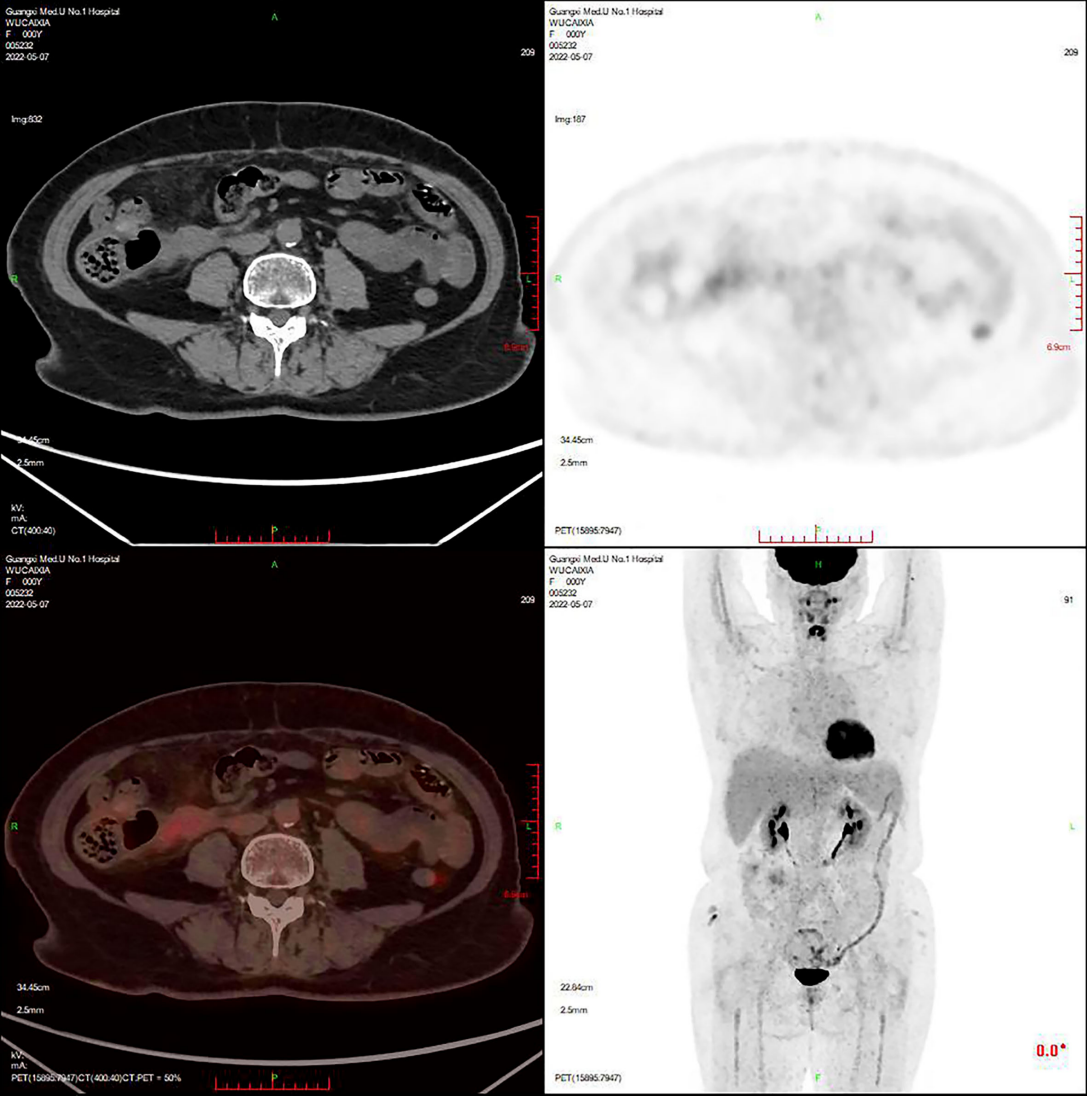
PET/CT. After chemotherapy and targeted treatment of lymphoma: (A) The right anterior lymph node lesions of the protodiaphragm have been absorbed and inactivated. (B) The primary ileocecal, colon, and mesenteric lesions were obviously improved; most of them were absorbed and inactivated, but tumor active tissues were still found in the ileocecal region. (C) No definite new lesions were found (Deauville score: 4 points).
